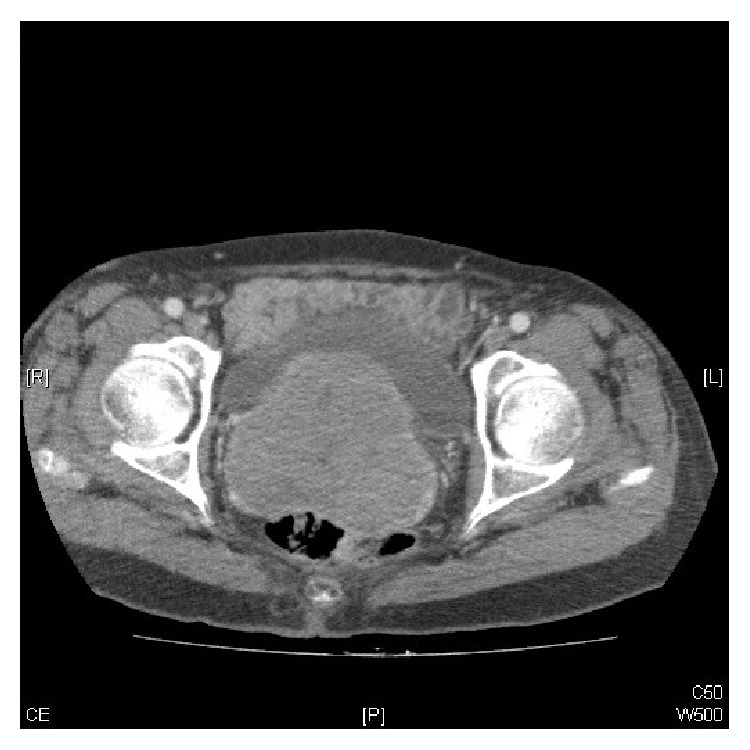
CT scan demonstrating a large mass occupying the vaginal cavity.
